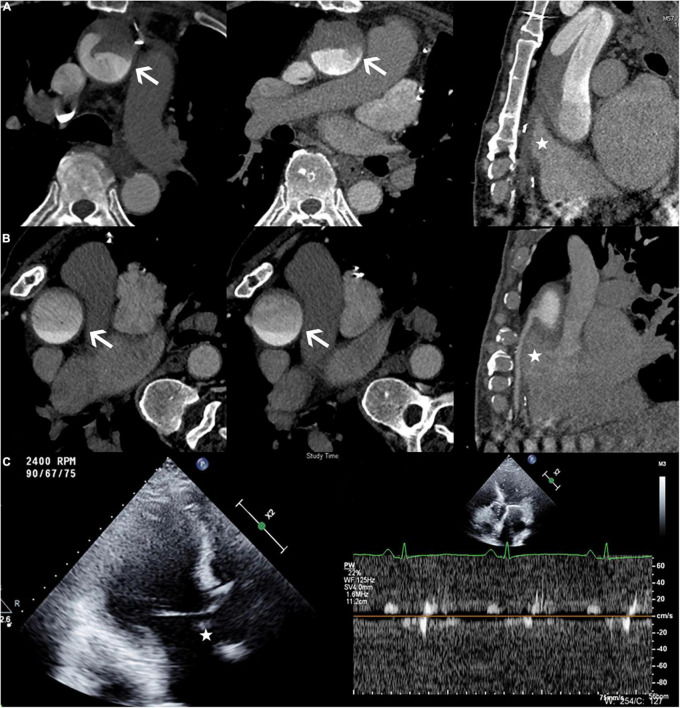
(A) Patient in the supine position. The axial view demonstrating an arc-shaped low-attenuation filling defect (white arrow) was seen in the root of the aorta, while the DAO was filling well. The multiplanar reformatted image from the CTA showed no contrast agent filling in the bypass of the right coronary artery (white star). (B) Patient in the right lateral decubitus position. The arc-shaped low-attenuation filling defect disappeared (white arrow), and the bypass of the right coronary artery filled well (white star). (C) The echocardiography (Philips EPIQ 7C; 45° reclining position; Three-chamber view) demonstrated the extremely low velocity of the aortic valve and no thrombus formation in the aortic root (white star).
